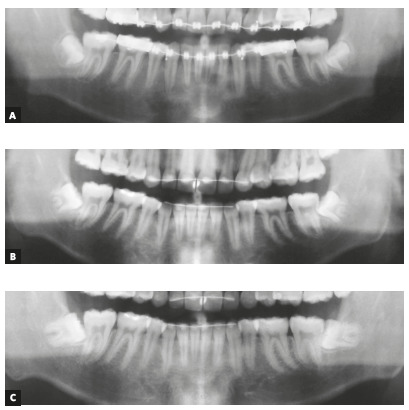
Panoramic radiograph at: A) the end of orthodontic treatment (PR1)
